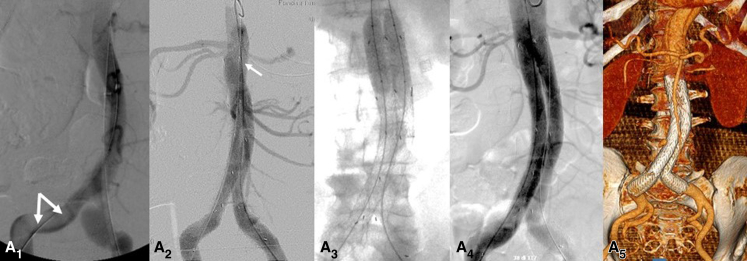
Intraoperative findings of endovascular repair of the infrarenal aorta. Preliminary angiography showing the dissecting flap (A_1_) (*white arrows*). Postendograft deployment control which showed the presence of an incomplete apposition of the endograft (A_2_) (*white arrow*). Reinforcement of the proximal edge of the endograft with kissing balloon expandable stent-grafting (A_3_). Final control with complete relamination of the lamella at the level of the renal arteries (A_4_). Six-month follow-up computed tomography angiography (A_5_).
